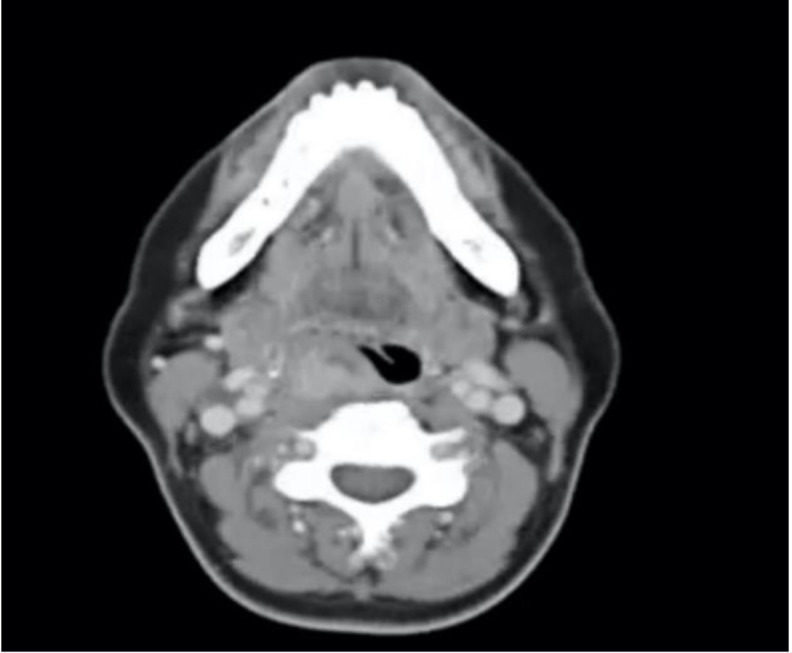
Axial CT image showing tumour at the right posterior pharynx
